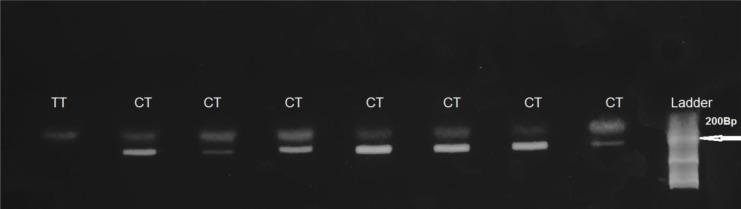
HinfI-treated MTHFR gene PCR fragments separated on 2% agarose gel. Presence of the C-allele resulted in no cleavage of the PCR product. Heterozygote C677T, (CT genotype) yielded two fragments of 198 bp and 175 bp. Homozygote C677T (TTgenotype) yielded two fragments of 175 bp.
